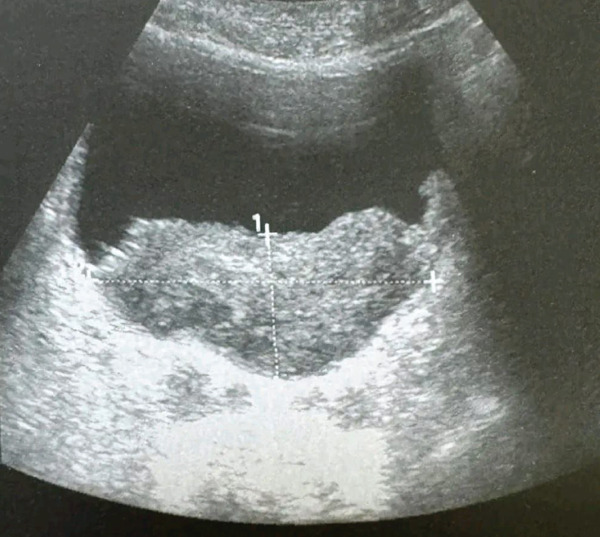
Transabdominal ultrasound of the urinary bladder.
